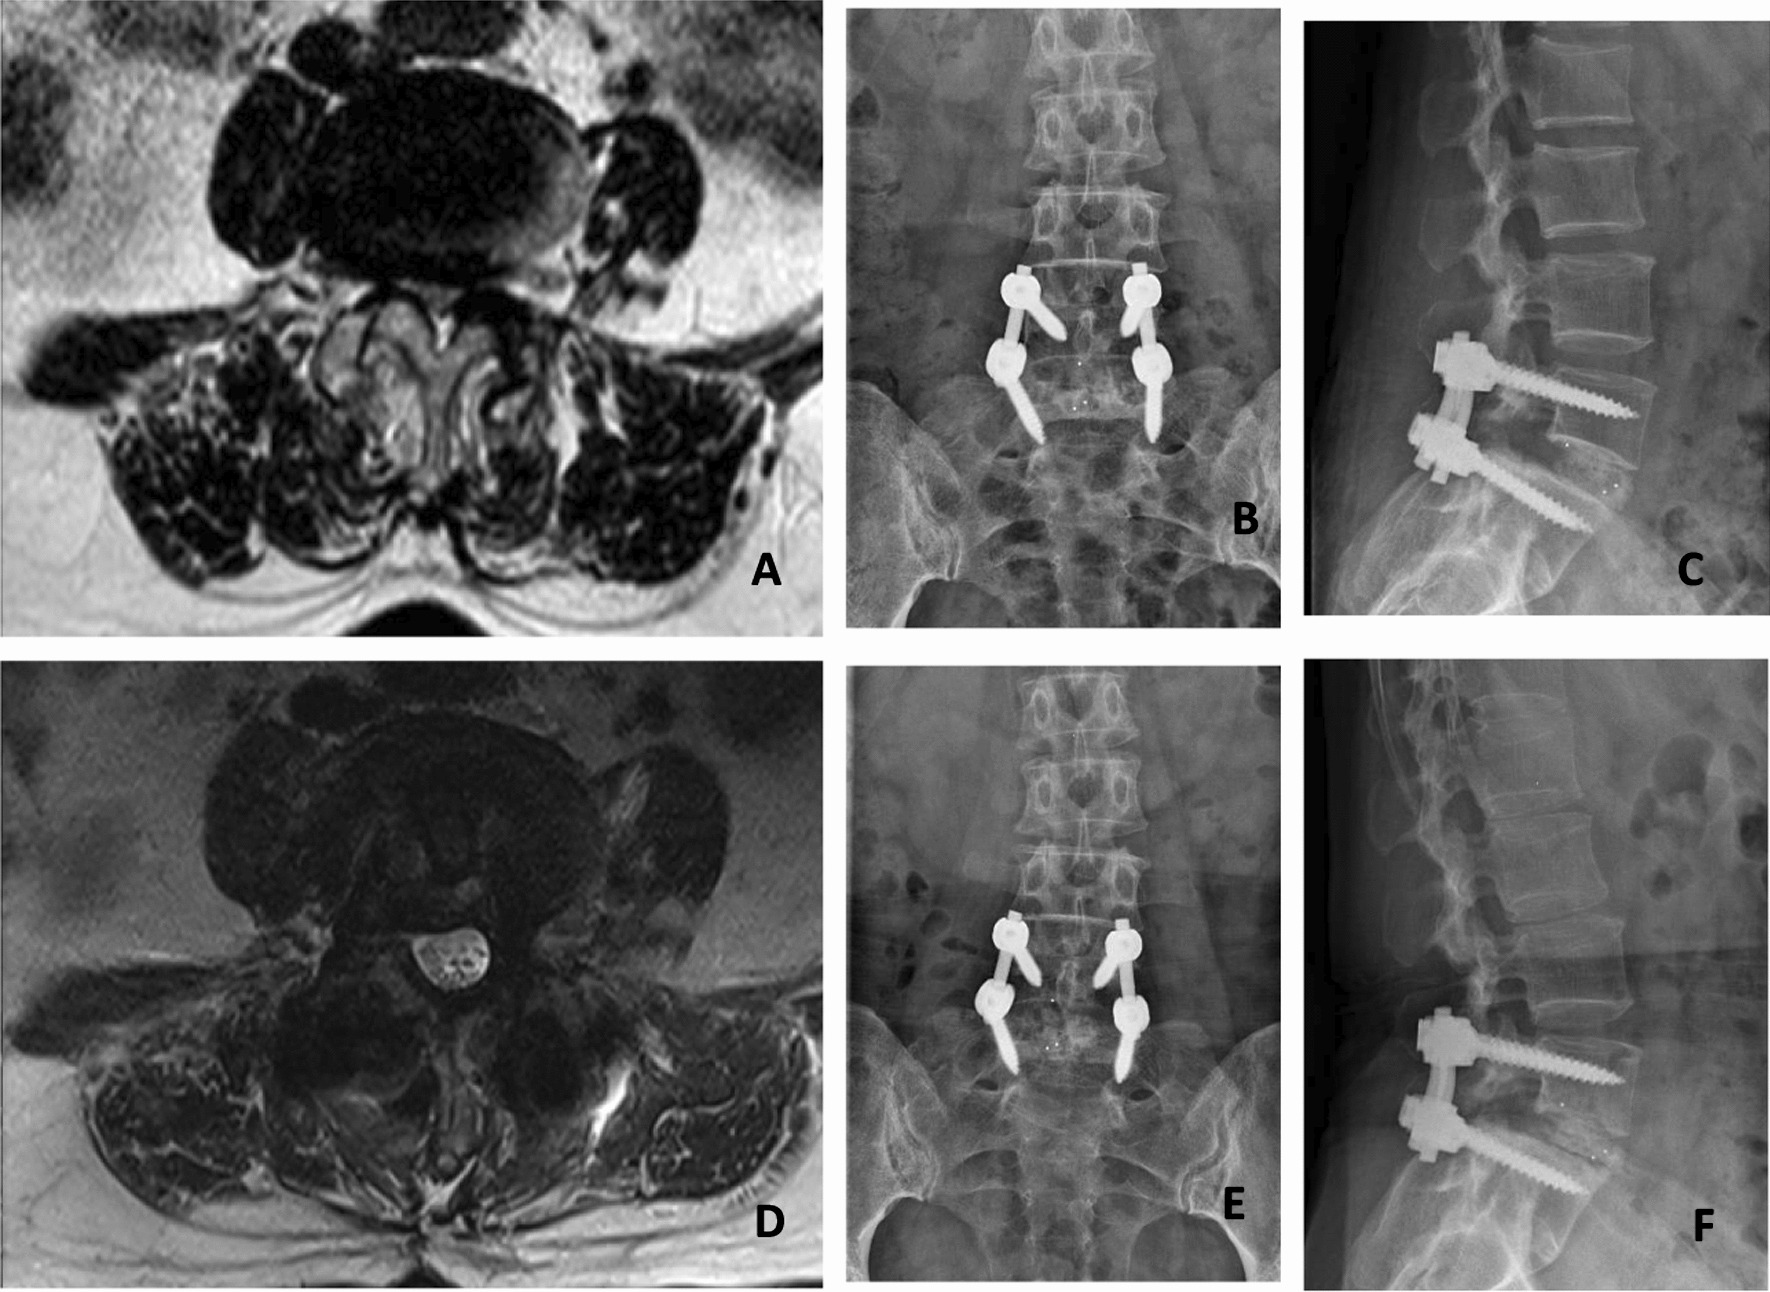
A 52-year-old woman complained of low back pain for more than 10 years, which was accompanied by right lower limb pain and numbness for 3 months. Before operation, axial MRI (A) showed lumbar disc herniation with lumbar spinal stenosis (L4/5). She underwent M-TLIF operation in our institution. The X-ray of anterior (B) and lateral (C) showed that the height of intervertebral space and intervertebral foramen returned to normal, and the position of interbody fusion cage was satisfactory. Axial MRI (D) showed that the spinal canal was significantly improved and the nerve root was decompressed completely. At the time of last follow-up, X-ray (E, F) shows that there is a clear and articular process fusion intervertebral fusion
